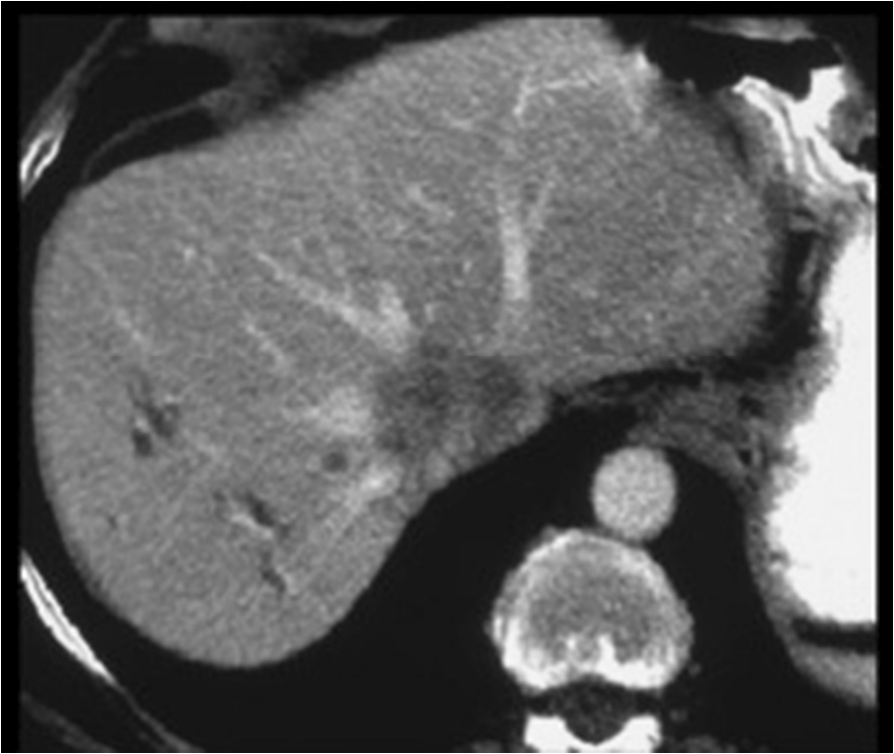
CT scan showing the liver metastasis infiltrating the inferior vena cava and all three liver veins. The tumor was mainly located in segment I.
